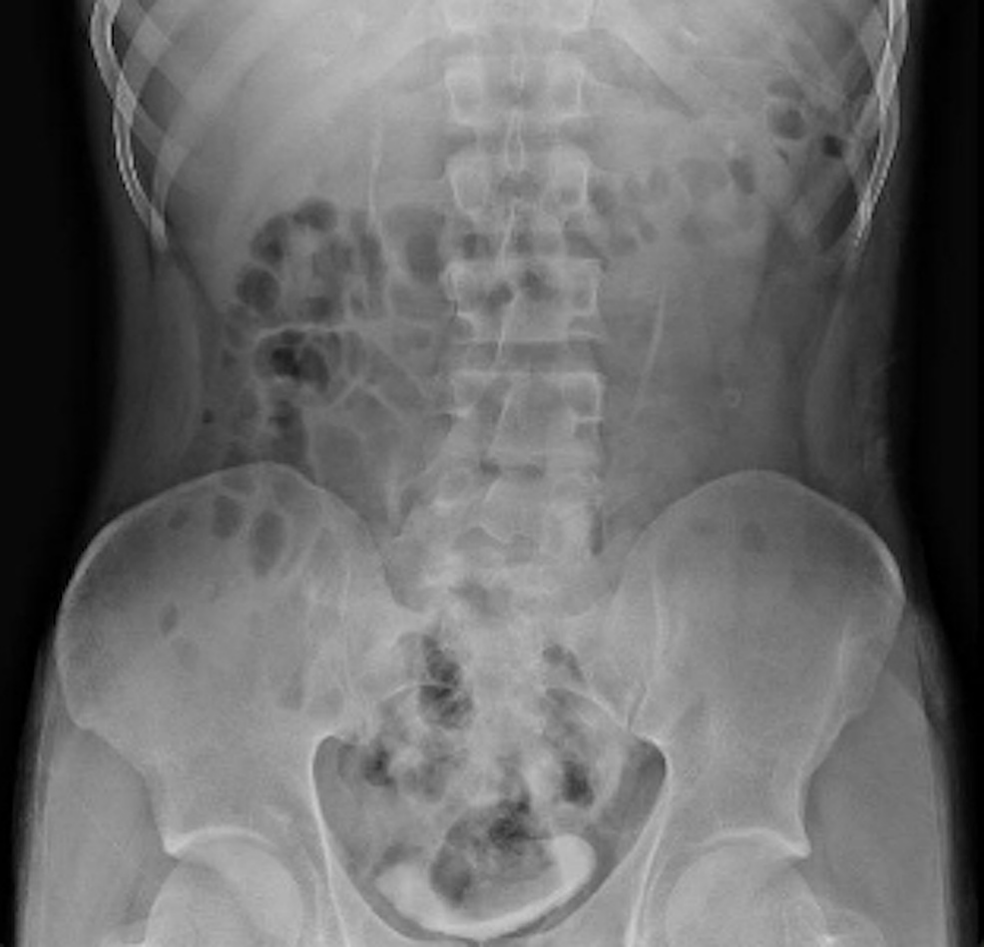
Unremarkable abdominal X-ray several hours after foreign bodies were seen on CT CT, computed tomography
